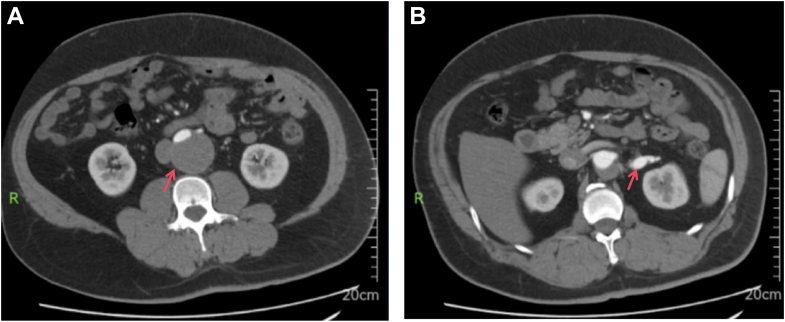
Preoperative Computed Tomography Angiography (A) Axial view showing chronic aortic dissection (Stanford Type B) extending to bilateral iliac arteries (arrow indicates compressed true lumen). (B) Axial view demonstrating aneurysmal dilation of left main renal artery (arrow).
